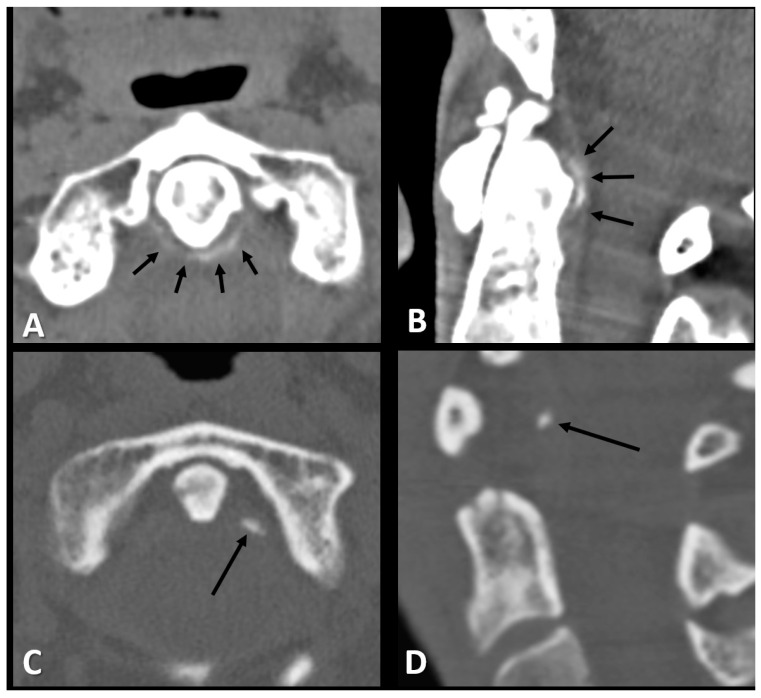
Axial and sagittal CT images of the periodontoid area of a 72-year-old woman (A,B) and a 52-year-old woman (C,D) with neck pain demonstrating the two types of periodontoid calcifications, linear (short arrows in (A,B)) and punctate (long arrow in (C,D)).
